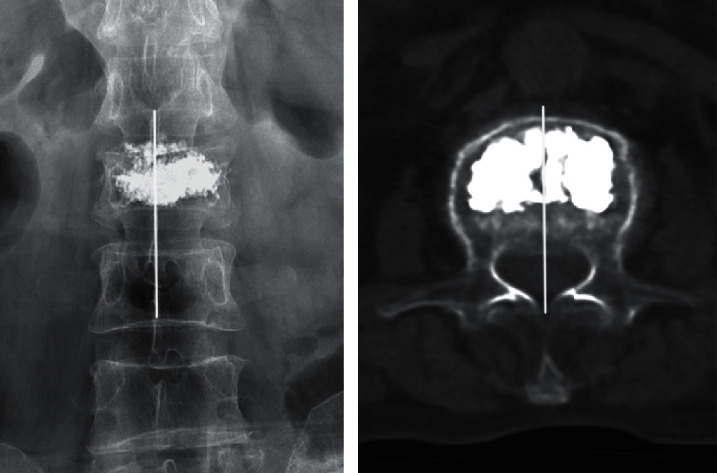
X-ray and CT showed that the distribution of bone cement crossed the midline with satisfactory diffusion.
